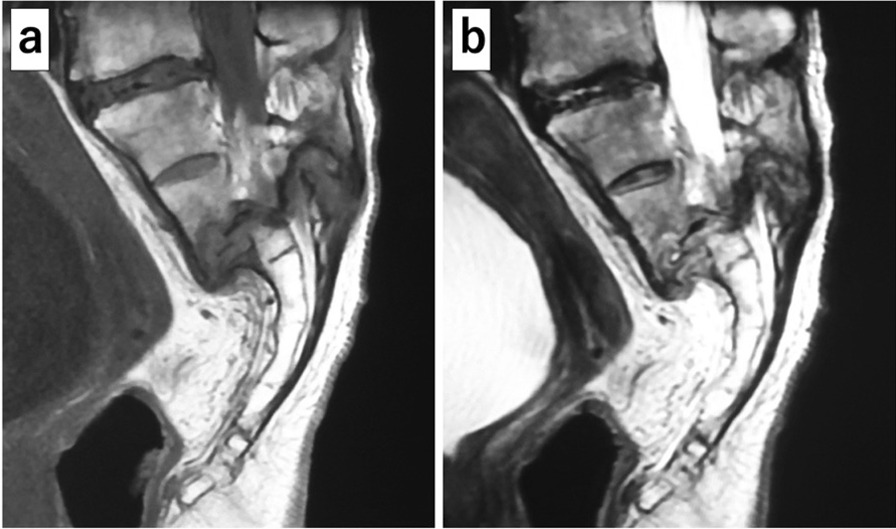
Preoperative MRI. a T1-weighted sagittal view, b T2-weighted sagittal view. Preoperative MRI showing severe stenosis of the spinal canal at the site of the transverse fracture
